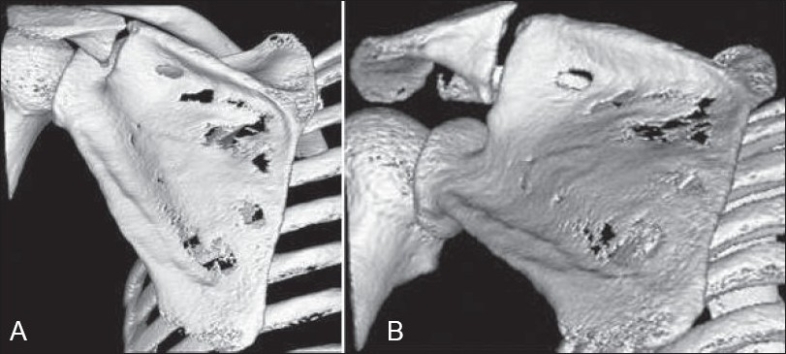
Three-dimensional reconstruction CT of the scapular spine fracture: (A) PA view and (B) oblique view
